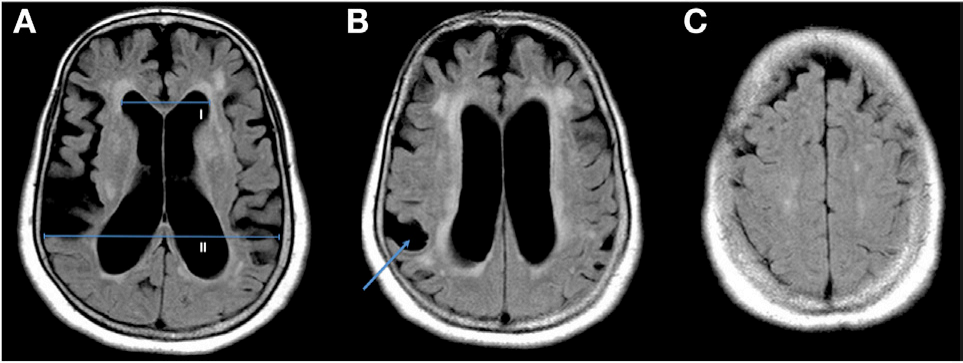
Shown are three axial slices of a cerebral MRI from a single non-shunted idiopathic normal pressure hydrocephalus patient with ventriculomegaly. The Evans index (EI) is calculated by dividing the maximal diameter of the frontal horns of the lateral ventricles [(A), I] by the maximal diameter of the skull [(A), II], values above 0.3 are considered hydrocephalic (20). Disproportionately enlarged subarachnoid space hydrocephalus features additionally consist of a focal enlargement of the cerebral sulci [(B), arrow] and tight high-convexity (C).
